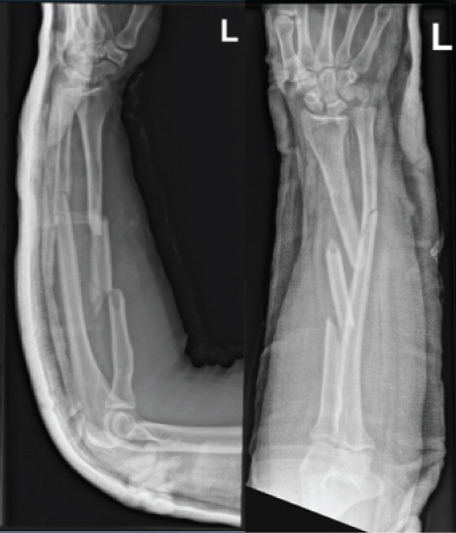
Forearm radiograph of the contralateral side.
